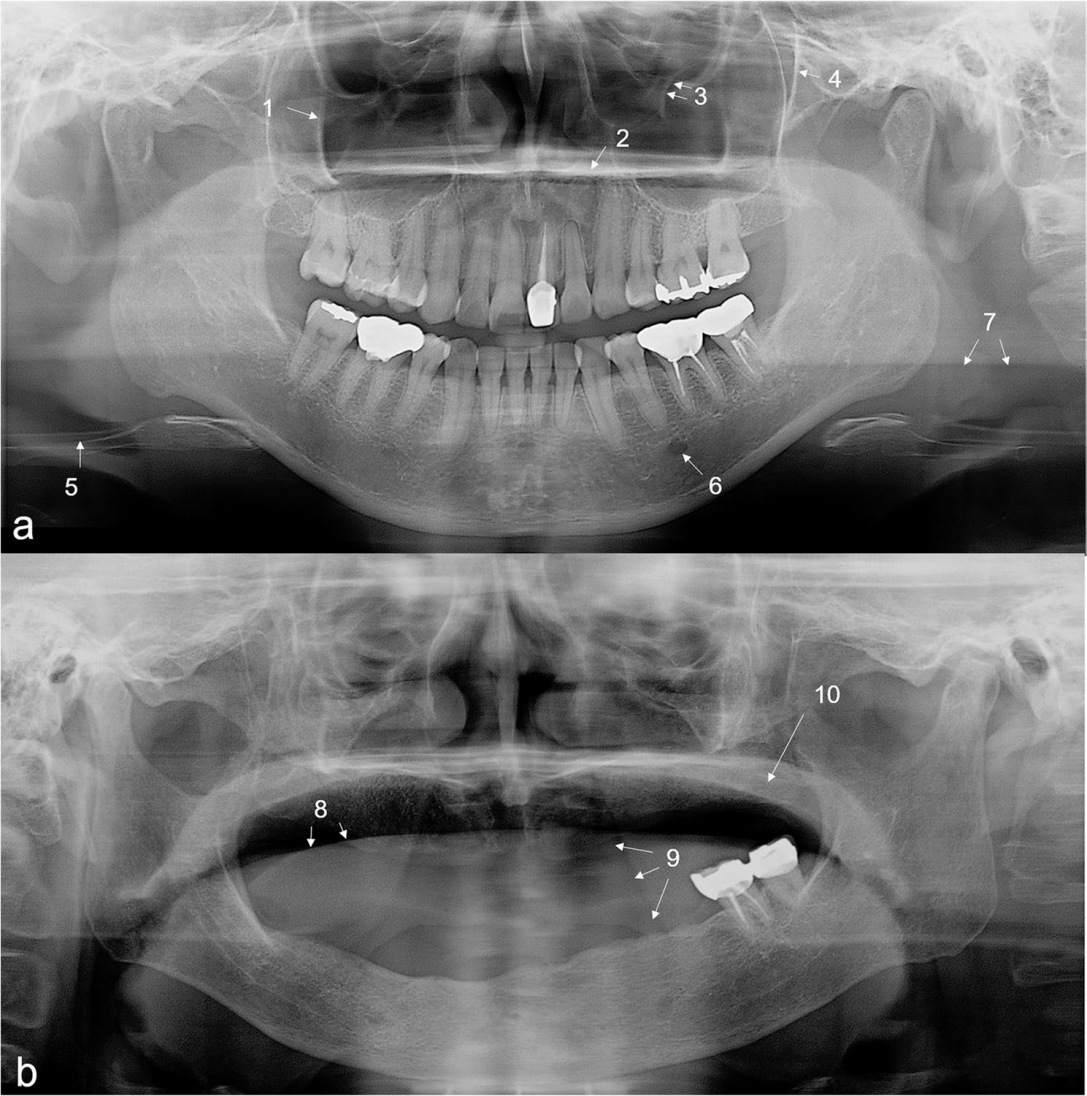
Ten questions about normal anatomical structures on two panoramic radiographs presenting bone (a) and soft tissue structures (b). 1: innominate line, 2: hard palate, 3: infraorbital canal, 4: pterygomaxillary fissure, 5: hyoid bone, 6: mental foramen, 7: ghost image of mandibular angle, 8: dorsal surface of tongue, 9: nasolabial fold, 10: soft palate
